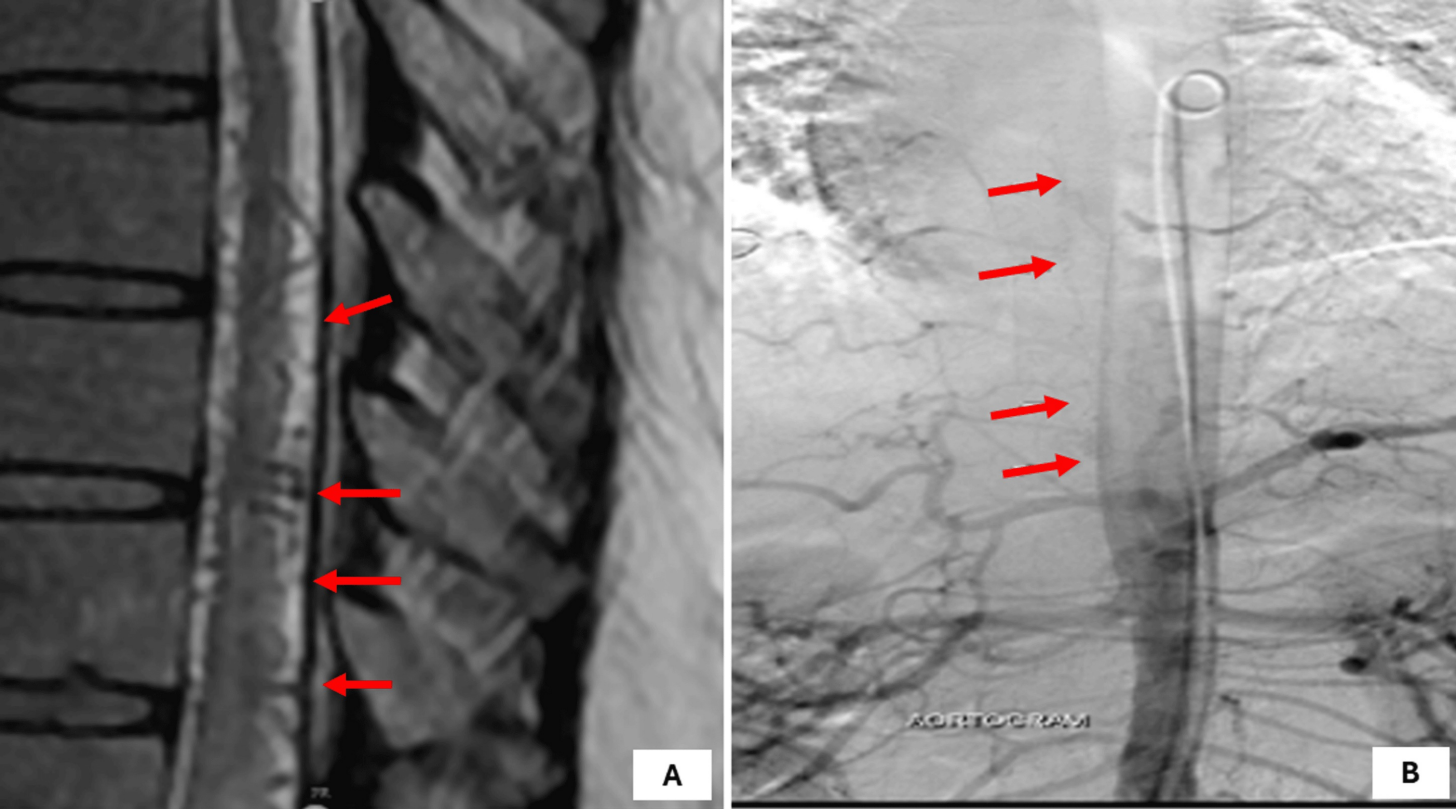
(A) Postoperative MRI and (B) DSA of the patient at one-year follow-up showing the recurrence of AVM AVM: arteriovenous malformation; DSA: digital subtraction angiography; MRI: magnetic resonance imaging
